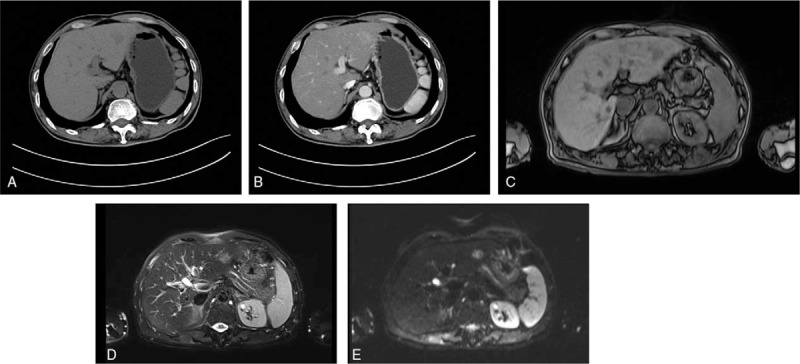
Computed tomography (CT) and magnetic resonance imaging (MRI) findings. Shown here is the mass with (A) low-signal intensity on plain CT and (B) slightly high-signal intensity on contrast-enhanced CT in the arterial phase. In contrast-enhanced MRI, the mass shows (C) low-signal intensity on T1-weighted imaging, and high-signal intensity on (D) T2-weighted imaging and (E) diffusion-weighted imaging.
